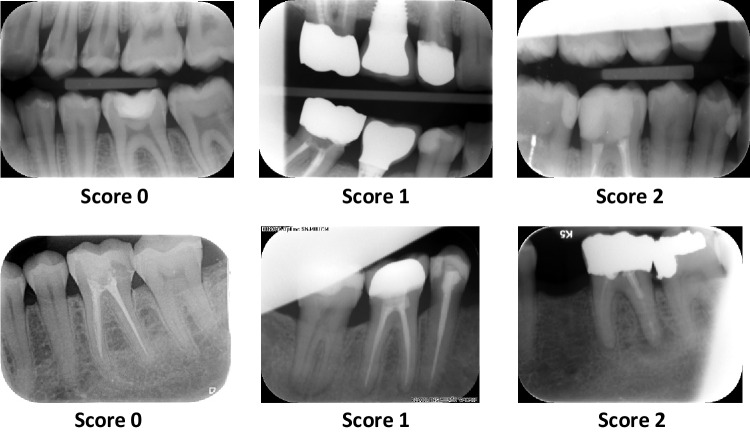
Example of scores 0, 1, and 2 on bitewing and periapical radiographs (based on score classification from Parrot and Ng [4]). Score 0: no cone cutting. Score 1: cone cutting that does not affect diagnostic value. Score 2: cone cutting that does affect diagnostic value
